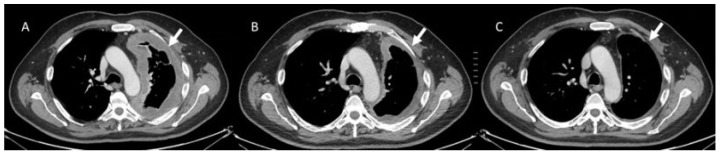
Complete response pattern in a mesothelioma patient treated with immunotherapy. CT scan assessment in pre-treatment phase (A) of lesion (arrow), at 3 months (B), and after 6 months (C).
